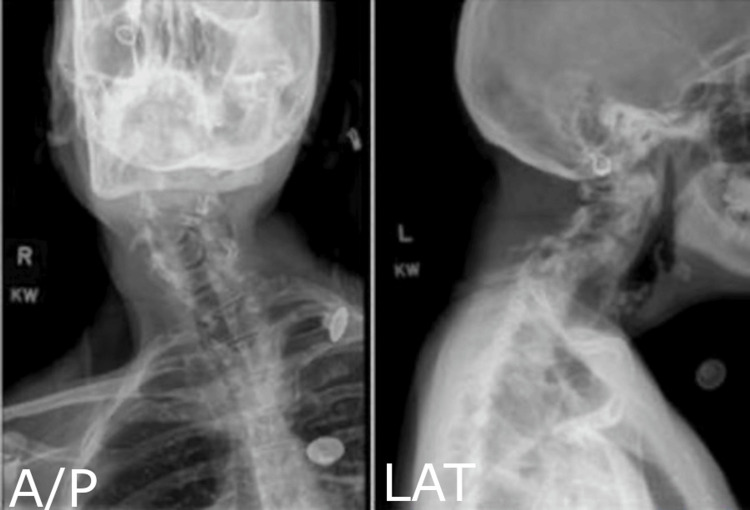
Preoperative Upright Cervical Radiographs Preoperative cervical radiographs demonstrating rigid apex-right scoliosis with a Cobb angle of 27 degrees, segmental kyphosis, C4-5 spondylolisthesis, with no deformity correction on supine A/P X-ray with axial traction. K Line (not shown) is negative. A/P: anteroposterior; LAT: lateral
